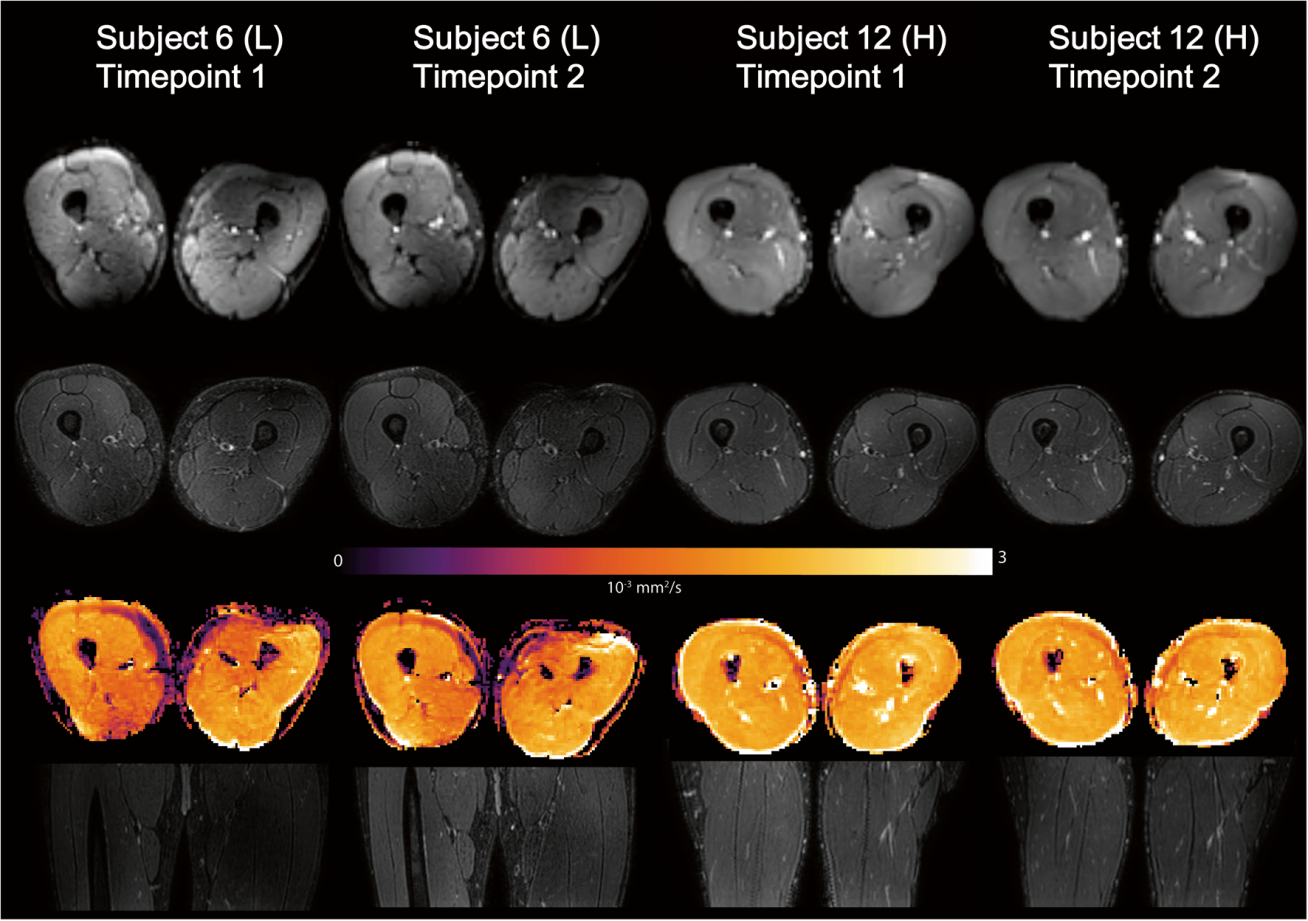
First and second time point scans of two subjects with (L) the lowest SNR and (H) typical high SNR. From top to bottom: axial DTI (*b* value = 0 s/mm^2^), T2-weighted images, MD map, coronal T2-weighted images. Mean SNR subject 6: time point 1, 24.8 ± 14.4; time point 2, 27.9 ± 13.4. Mean SNR subject 12: time point 1, 47.6 ± 11.5; time point 2, 54.2 ± 14
